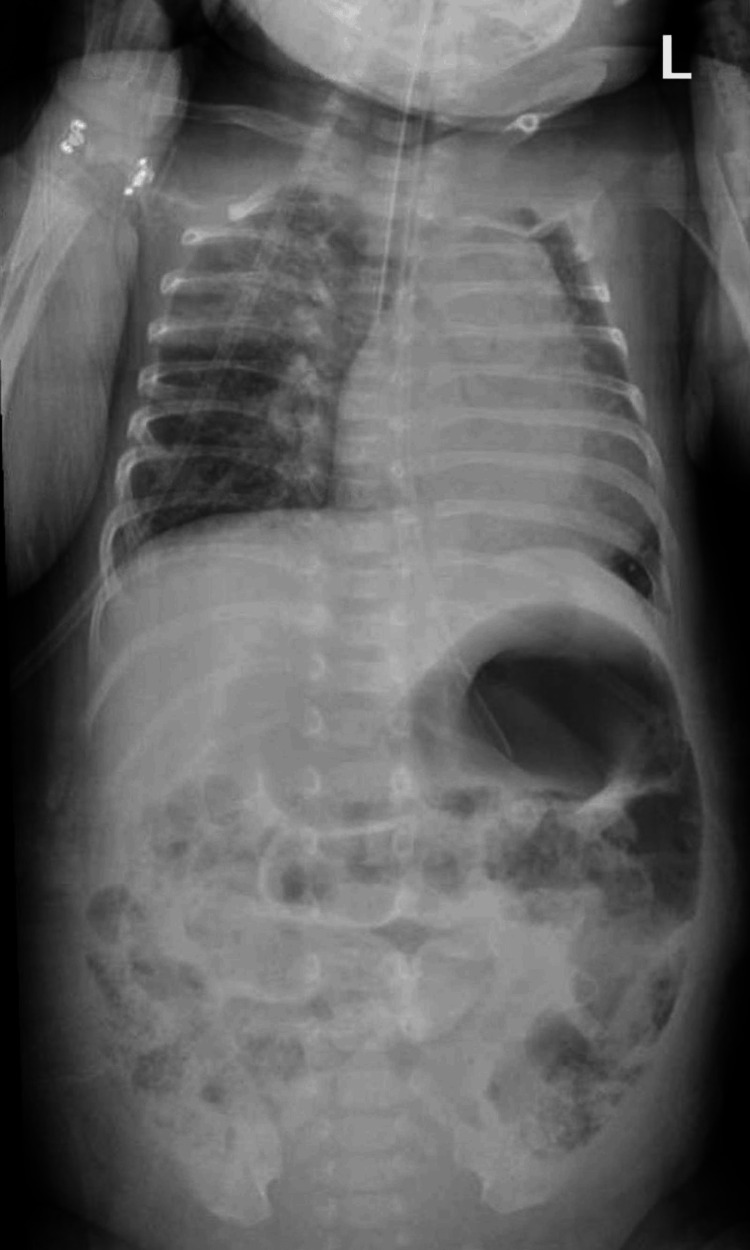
X-ray showing findings consistent with necrotizing enterocolitis (NEC)
